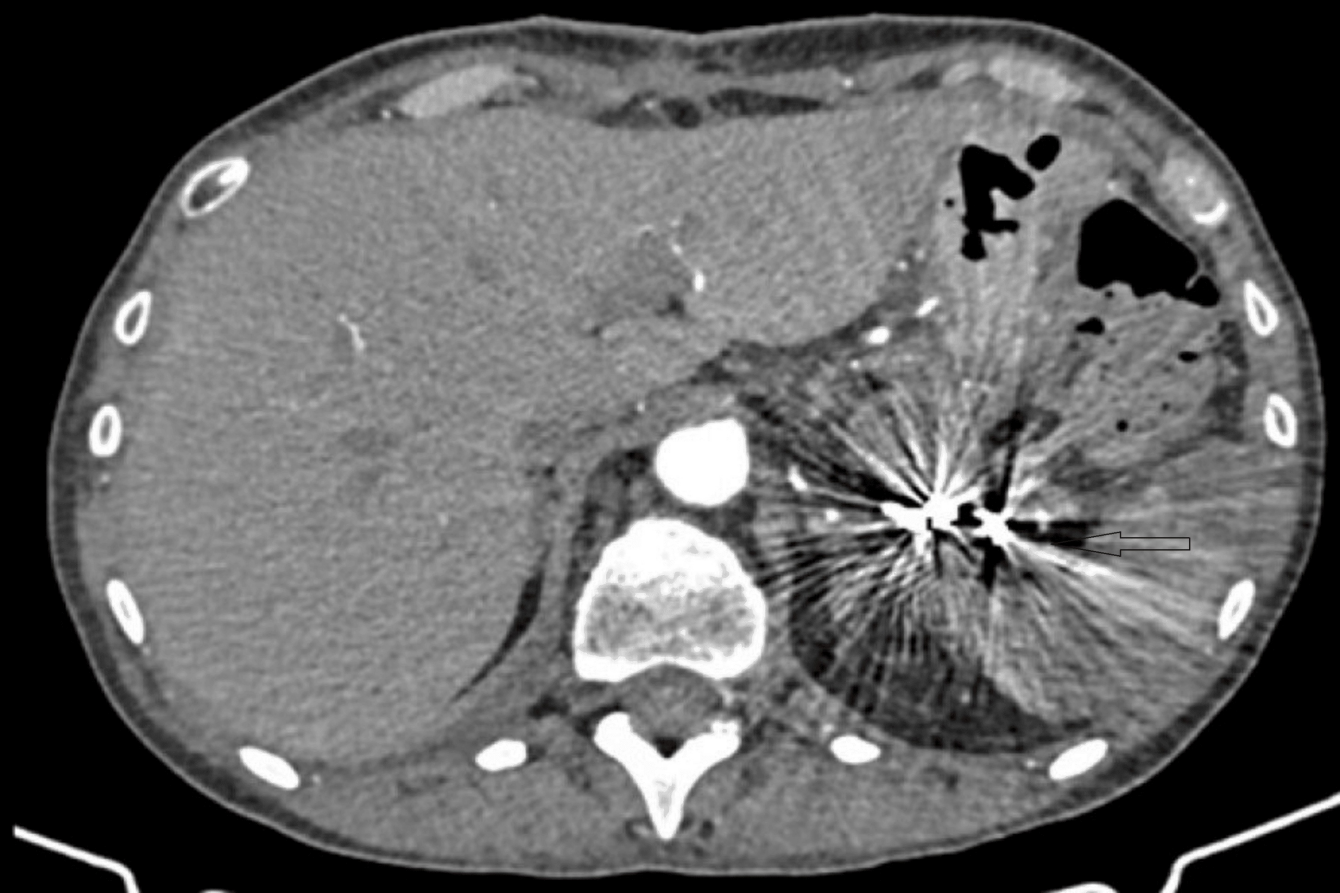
CTA performed after the embolization of the splenic artery, demonstrating total occlusion of the pseudoaneurysm CTA: CT angiography
